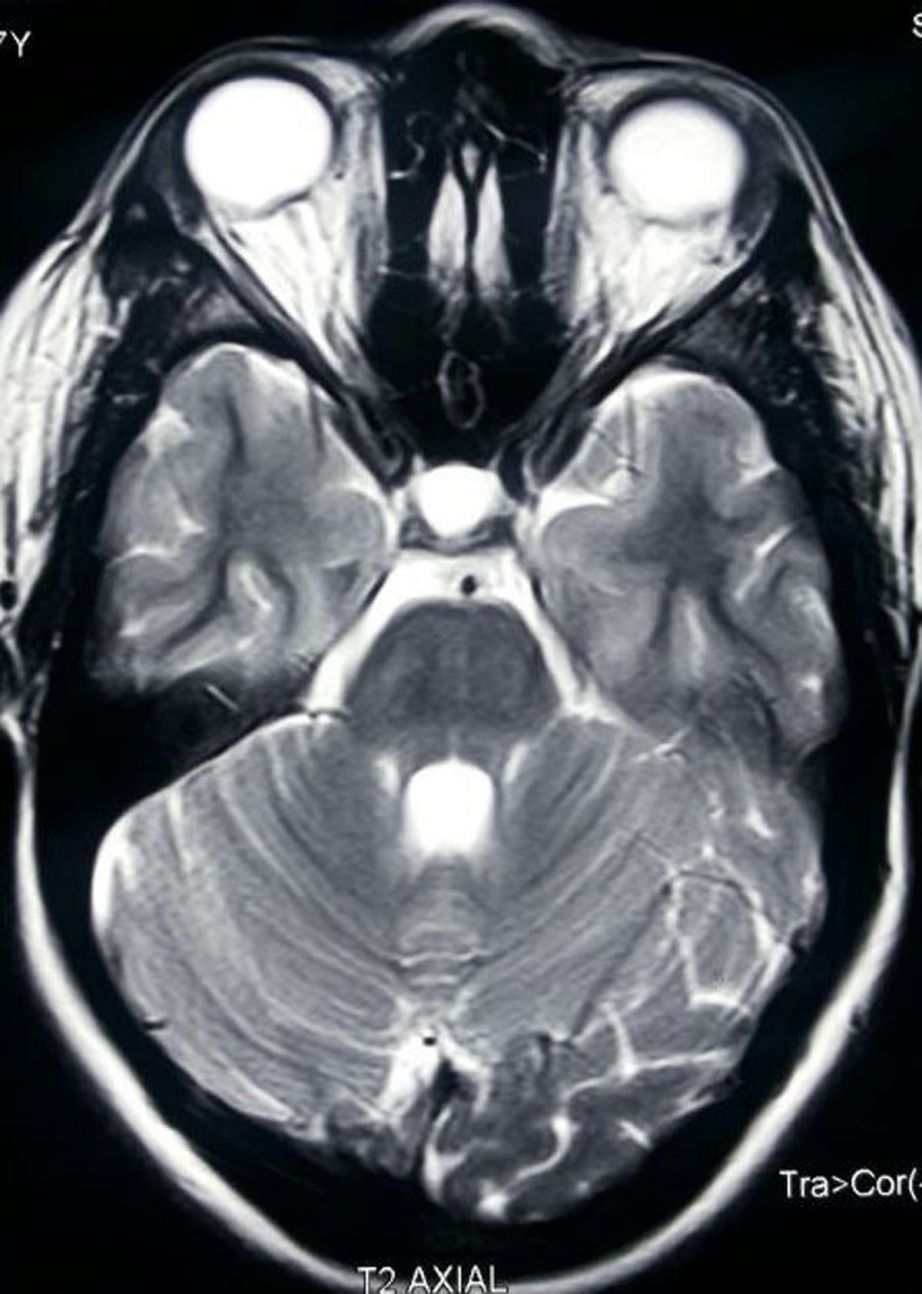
MRI Brain T2W axial image showing empty sella and flattening of posterior aspect of globe.
